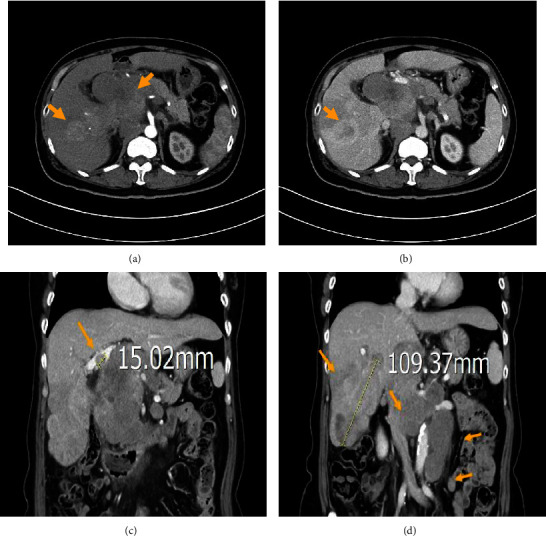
Triphasic abdominal computed tomography scan findings: The axial view revealed multiple tumor mass lesions in both the lobes, with arterial phase hyperenhancement (a) and late phase hypodensity (b). The coronal view revealed tumor thrombi in the portal vein (c) and tumor thrombi in the hepatic vein (d). The coronal view revealed maximum size of these tumor mass lesions was about 10.9 cm at S5/S6 (d). The coronal view revealed multiple enlarged lymph nodes in the paracaval and para-aortic regions of the retroperitoneum (d).
